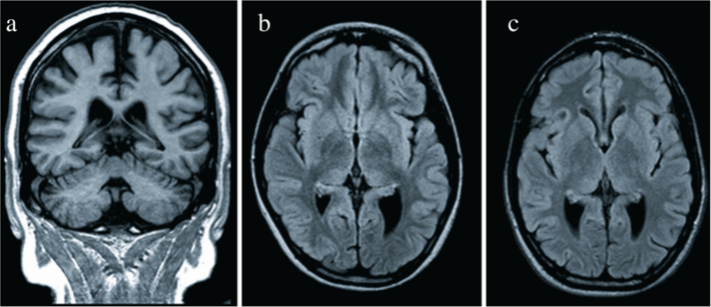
Brain MRI: coronal T1-weighted (a) and axial FLAIR (b, c) images of Alström syndrome patients. a) 31-year-old male: mild brain atrophy with enlargement of ventricles and subarachnoid spaces; b) 11-year-old girl: enlargement of the left atrium of lateral ventricle; c) 18 year-old boy: bilateral dilated atria of the lateral ventricle.
